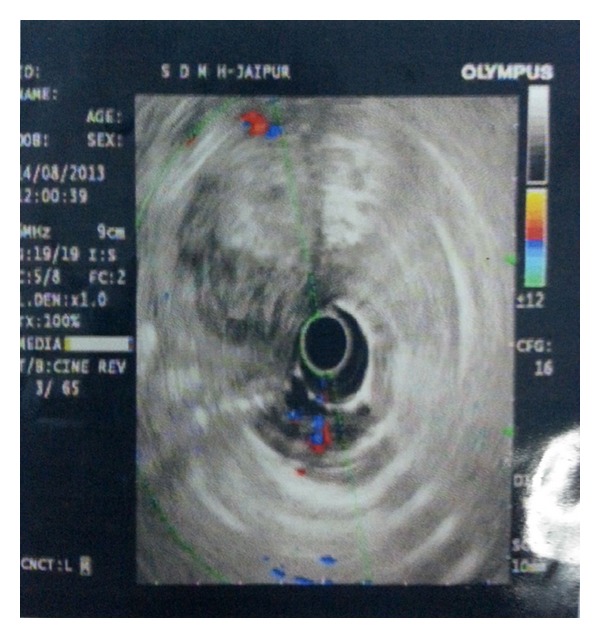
EUS Doppler depicting a mass in wall of stomach.
